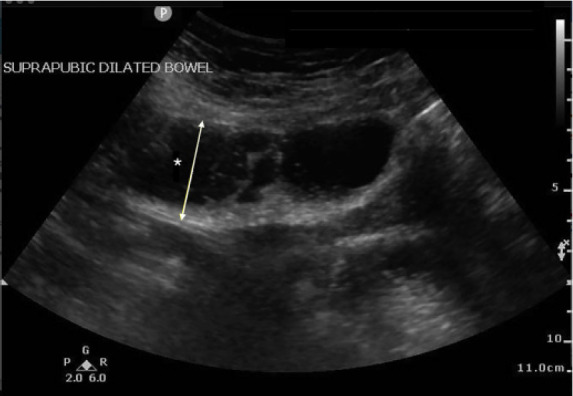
Point-of-care ultrasound demonstrating long-axis view of dilated, fluid-filled loops of bowel (*) measuring 4.1 centimeters from outer bowel wall to outer wall, consistent with small bowel obstruction.
